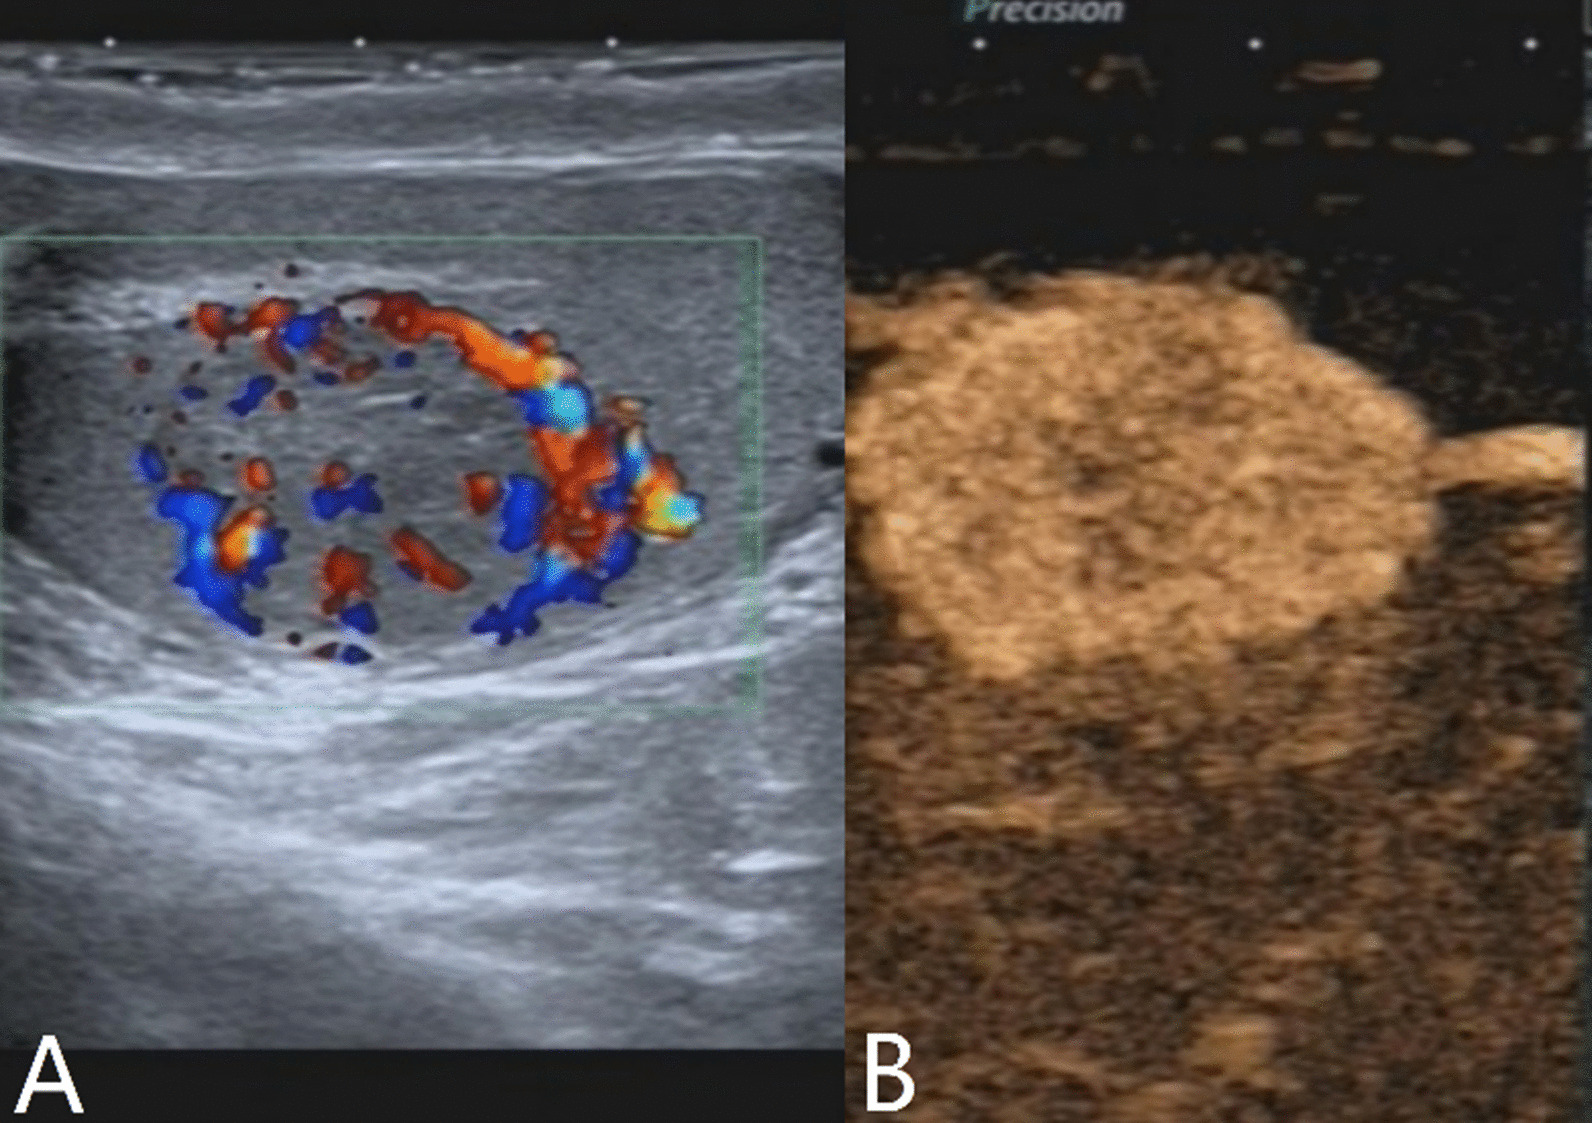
Testicular Leydig cell tumor. A Conventional ultrasonography showed that the right testis had a clear isoechoic boundary with abundant blood flow and peripheral annular blood flow. B Contrast-enhanced ultrasonography showed uniform high enhancement
